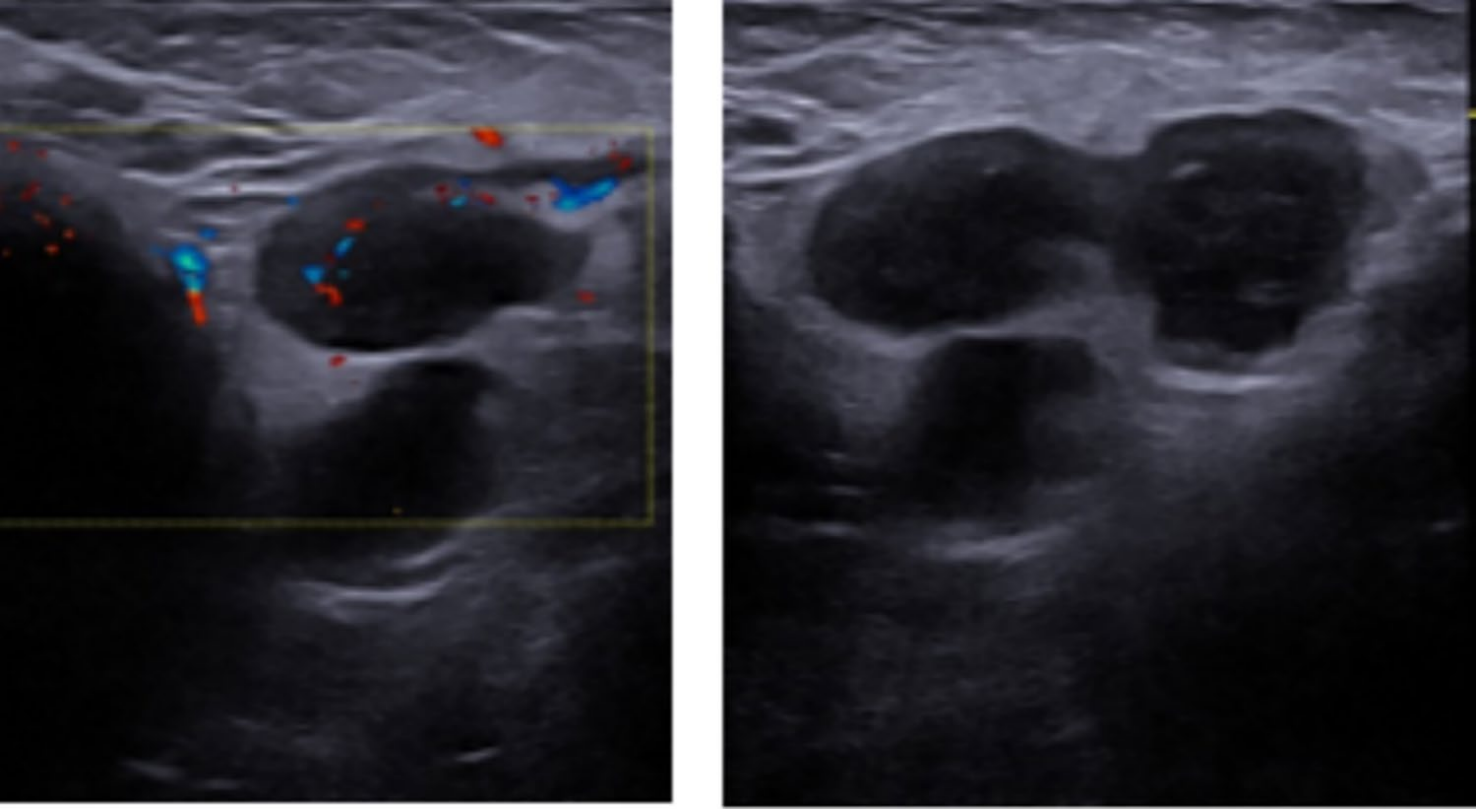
Ultrasound analysis at presentation
